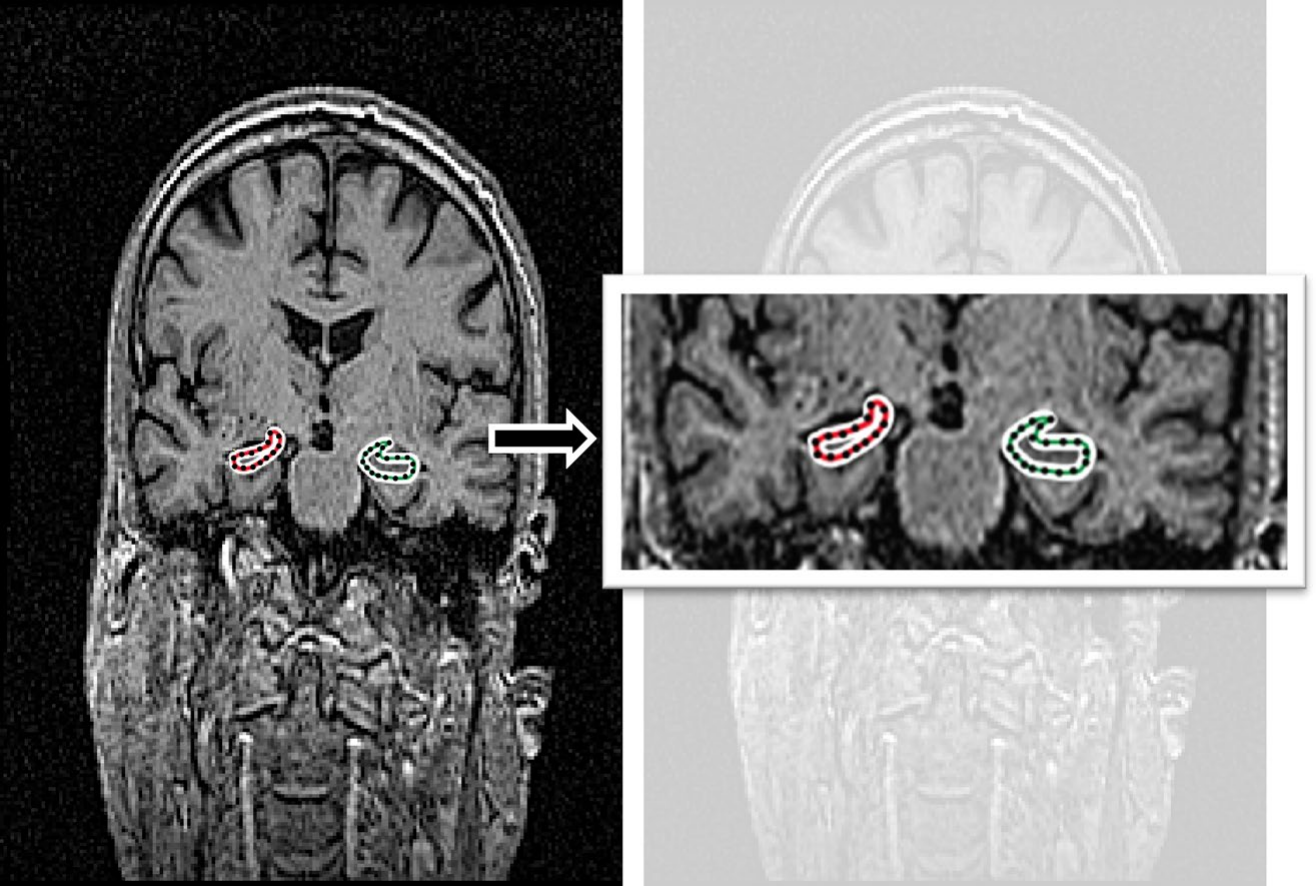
Exemplary labeling images in the AD class with sample MRI images sourced from the Alzheimer’s Disease Neuroimaging Initiative (ADNI) dataset.
